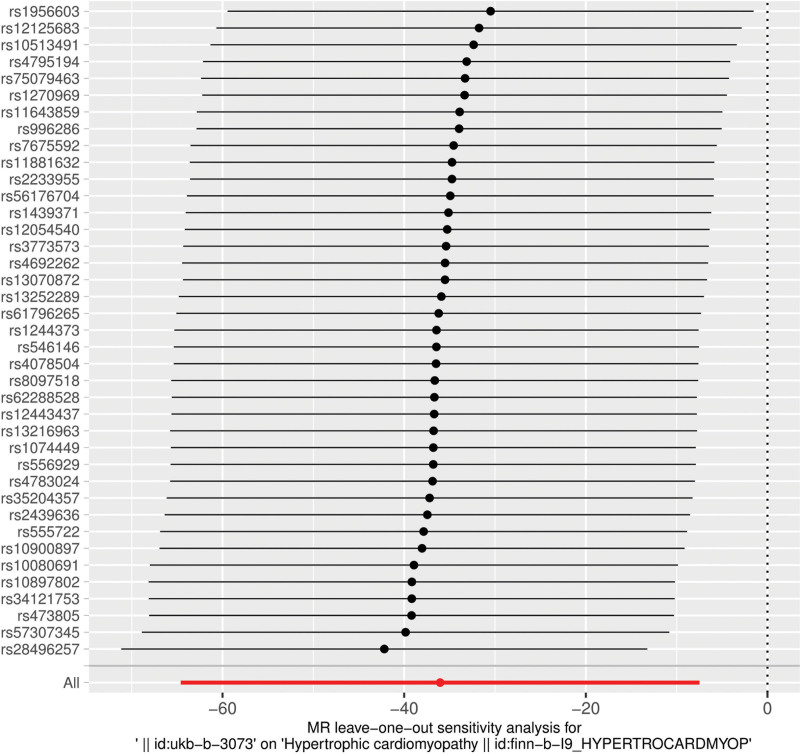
Leave-one-out sensitivity analysis. The plot visualizes the stability of the overall MR estimate for the association between CT scanning propensity and HCM risk by iteratively removing each individual SNP from the analysis. CT = computed tomography, HCM = hypertrophic cardiomyopathy, MR = Mendelian randomization, SNP = single-nucleotide polymorphism.
